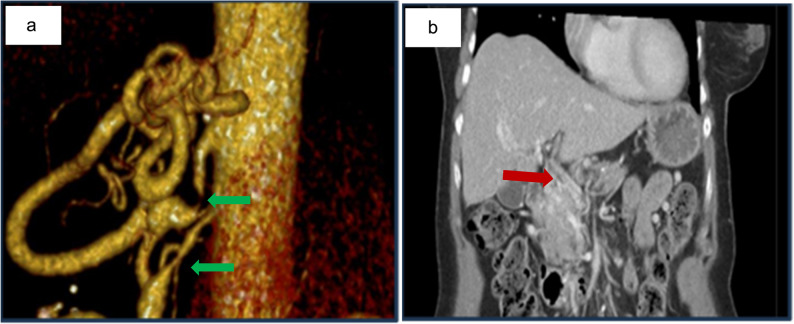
a shows a reconstructed CT image revealing high-grade stenoses of the celiac trunk and superior mesenteric artery (green arrows) with a dilated Riolan anastomosis and b shows portal vein thrombosis post-splenectomy in a 71-year-old woman with idiopathic splenic vein stenosis
